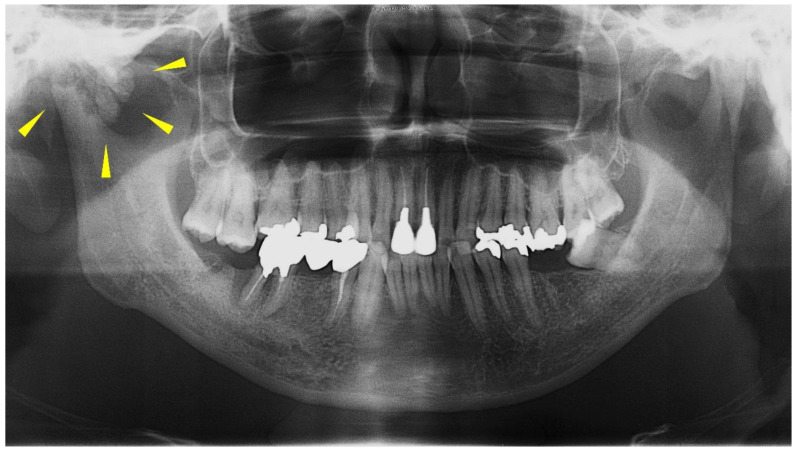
Panoramic radiograph from the first visit. The ill-defined calcification around the mandibular condyle is shown (yellow arrows).
